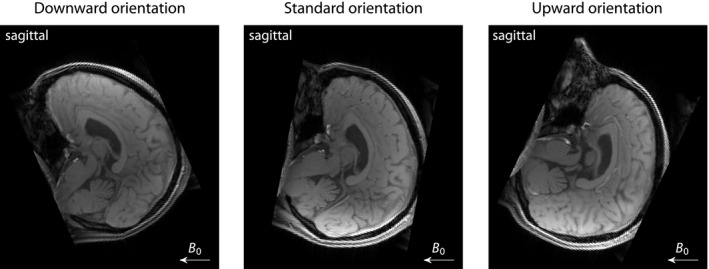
The gradient‐echo measurements were carried out at 3 different head orientations (downwards, standard, upwards) with respect to the external magnetic field. The rotation axis of the head inside the MRI scanner was left‐right; the angle between upward and downward head position was
